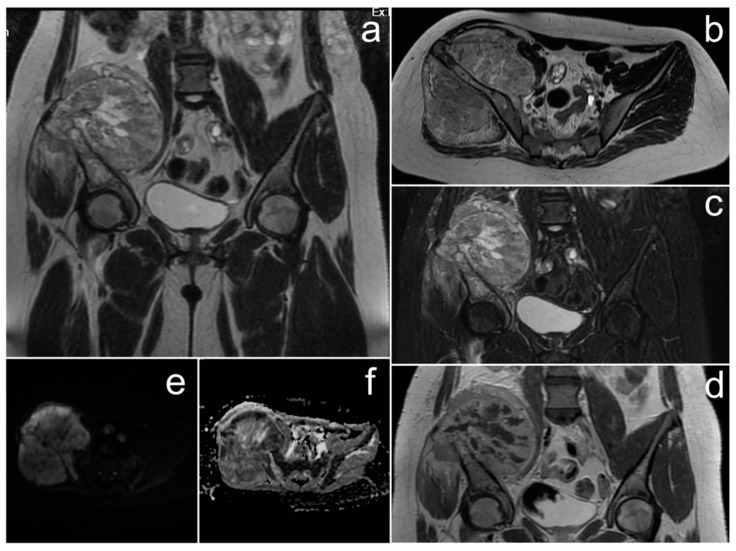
A 13-year-old girl with Ewing sarcoma of the right iliac wing with a giant soft tissue tumor on both sides of the bone (a–f). (a,b)—FSE/T2; (c)—STIR; (d)—post-contrast FSE/T1; (e)—DWI; (f)—ADC map.
